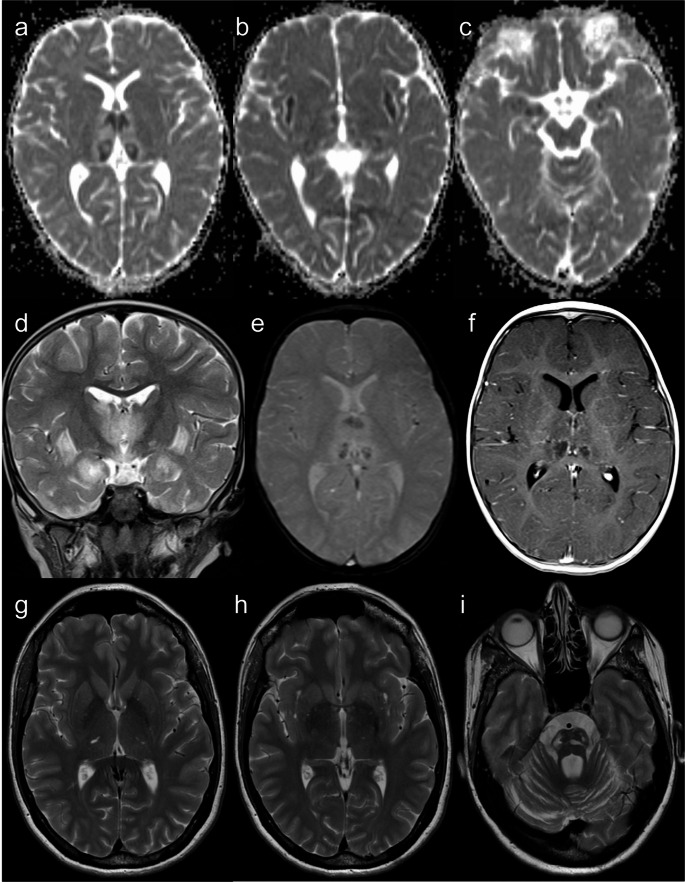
Familial acute necrotizing encephalopathy. A 13-month-old male patient with status epilepticus, preceded by fever and lethargy. His brain MRI (a to c – axial ADC maps, d – coronal T2, e – axial SWAN, and f – gadolinium-enhanced axial T1) demonstrates symmetrical T2-hyperintense lesions in the thalami, external capsules, and hippocampi. These lesions exhibit low signal on ADC maps, hemorrhagic foci, and thin peripheral enhancement. Mass effect is noted, leading to compression of the third ventricle and the temporal poles of the lateral ventricles. Overall, these findings are consistent with ANE. The patient’s mother had an episode described as corticosteroid-responsive encephalomyelitis with elevated anti-Influenza B antibody titers at 12 years of age. Her brain MRI at 40 years of age (g and i – axial T2) shows cavitated lesions in the thalami, sublenticular regions, external capsules, and ventral pons bilaterally, accompanied by secondary diffuse cerebellar atrophy. These findings are compatible with sequelae of ANE. Genetic testing confirmed the presence of RANBP2 gene mutations in both the patient and his mother
